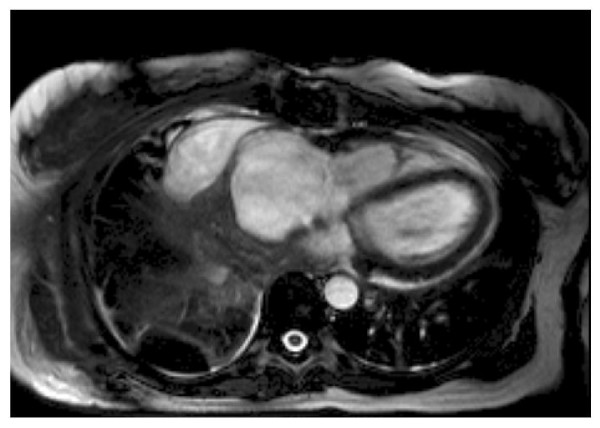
Magnetic resonance image obtained during post-operative surveillance, showing no recurrence of the tumour.
